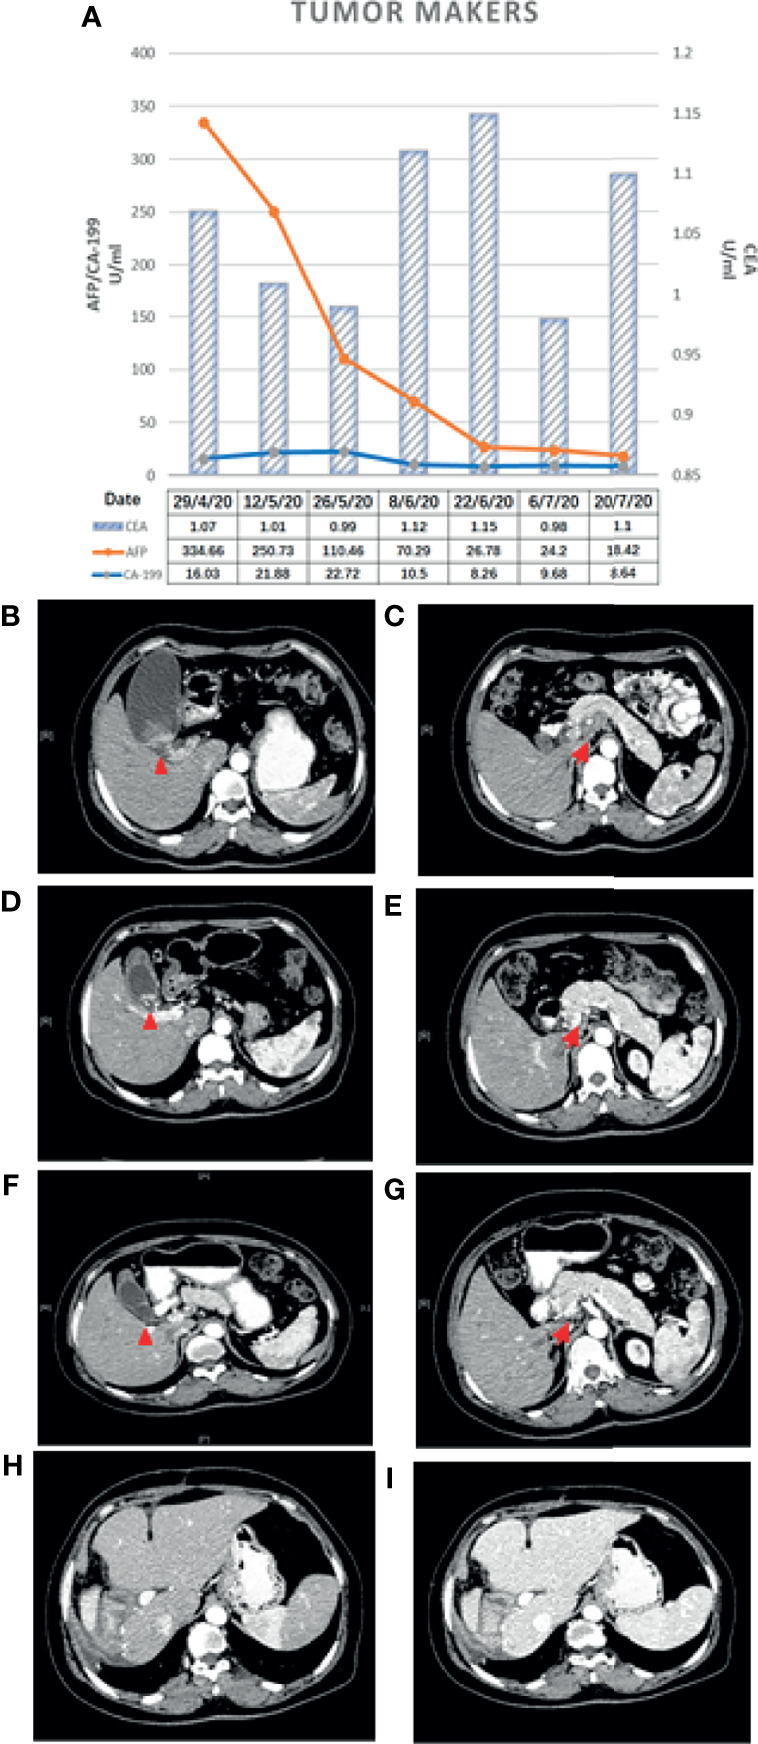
(A) Changes in the tumor makers. (B, C) Baseline CT scan. (D, E) CT scan after the first treatment cycle. (F, G) CT scan after the second treatment cycle. Changes in CT findings: the primary gallbladder cancer and metastatic lymph nodes shrank after treatment with gemcitabine + albumin-bound paclitaxel combined with camrelizumab for two cycles. (H, I) CT examination after surgery. No recurrence or metastasis was found on abdominal CT at 14 months. The *red triangle* denotes the primary lesion and the *red arrow* indicates the metastatic lymph nodes posterior to the pancreas.
